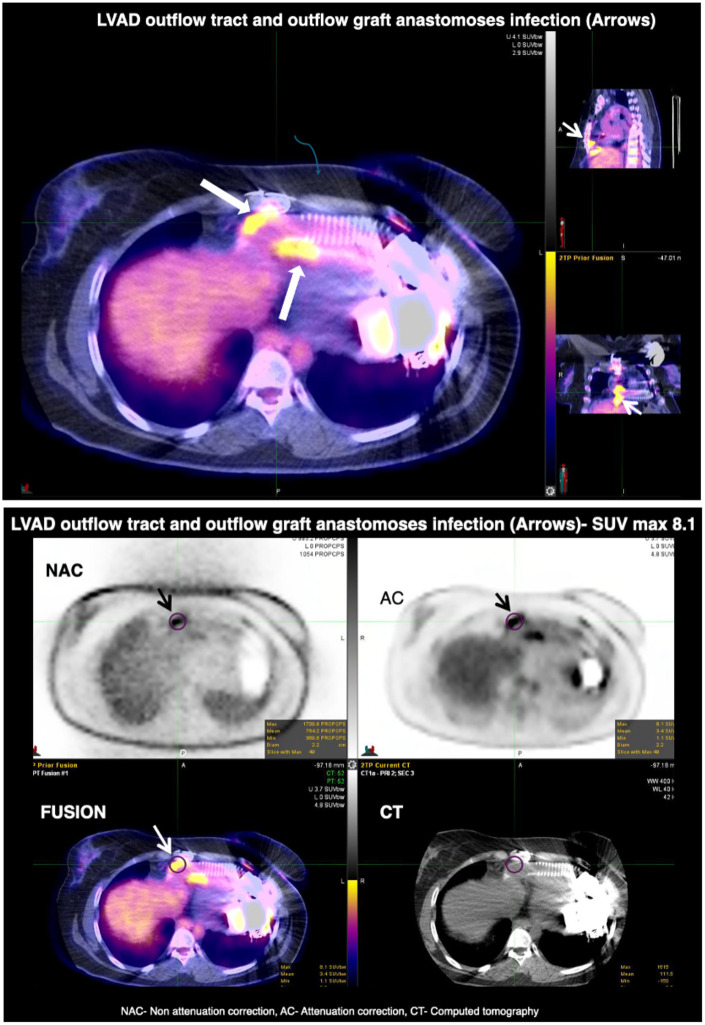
FDG-PET images of LVAD outflow tract and outflow graft anastomoses infection.
